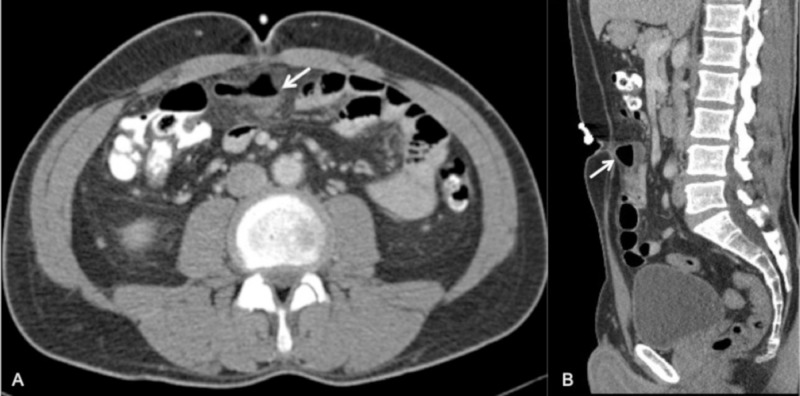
Axial (a) and sagittal (b) post-IV contrast-enhanced imaging demonstrating a gas- and fluid-filled mesenteric collection (arrows) located deep to the recently pierced umbilicus. IV - Intravenous, CT - computed tomography
